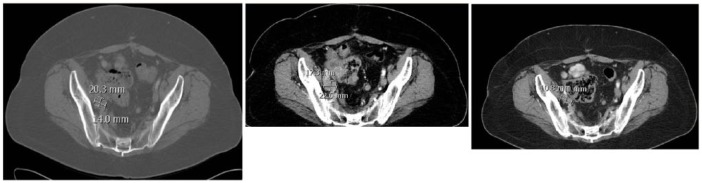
Computed tomography scans, from left to right, showing a pelvic target lesion (1) at baseline measuring 20.3 mm largest diameter, (2) after 1 month of RRx-001 treatment measuring 22.6 largest diameter, and (3) after 2 cycles of carboplatin-taxane measuring 10.8 mm largest diameter.
